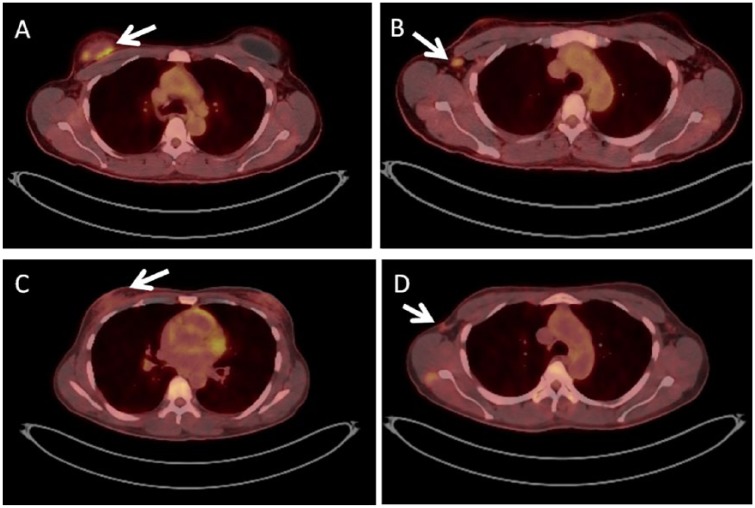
Axial PET scans. A and B were acquired prior to chemotherapy, while C and D were acquired after 6 cycles of CHOP. (A) Demonstrates hypermetabolic soft tissue densities around the breast implant, while (B) shows a hypermetabolic right axillary lymph node. (C and D) Show residual FDG-avidity in the right chest and axilla, respectively.
